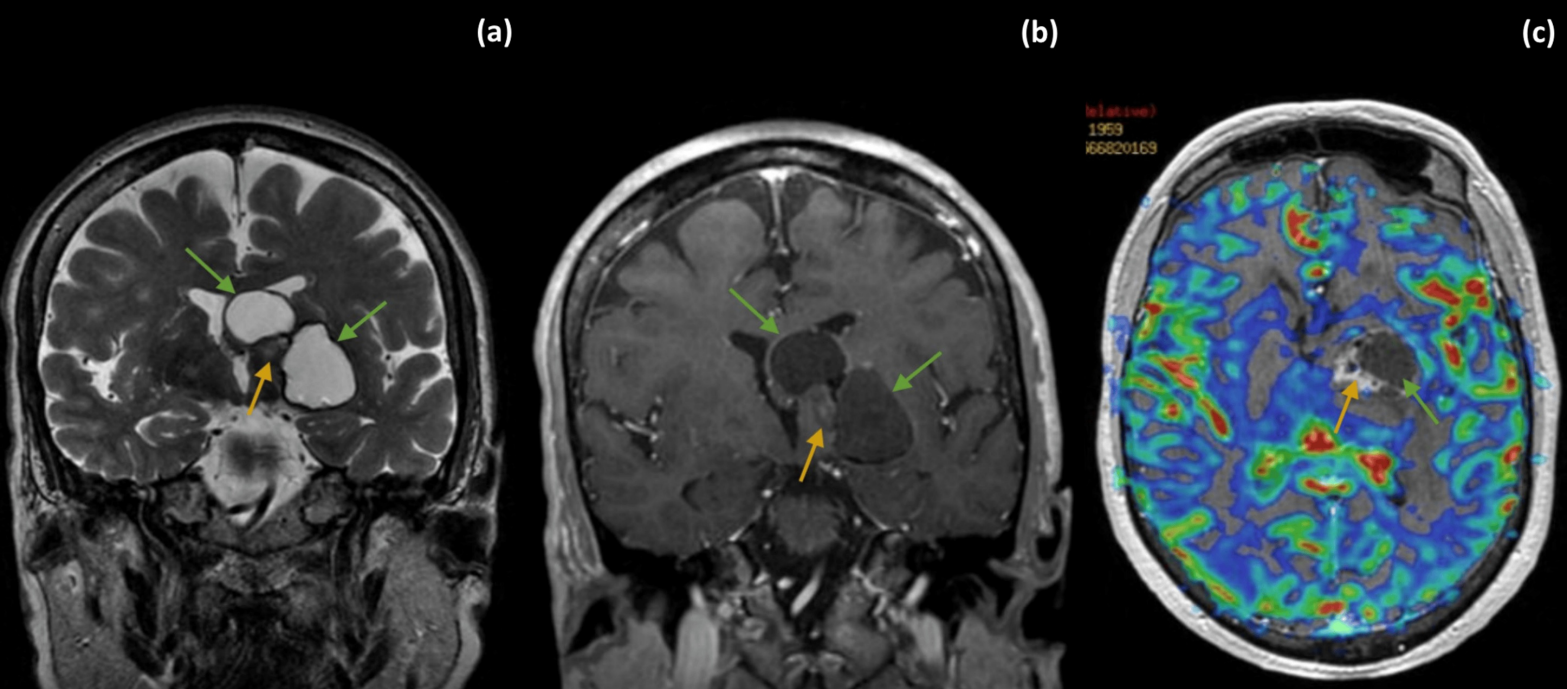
Diagnostic brain MRI. Brain MRI showed a left-side thalamic lesion with one solid and two large cystic components, one extending on the left lenticulocapsular region and the other encroaching to the body of the left lateral ventricle. The two cystic components (green arrows) were homogeneously hyperintense on T2-WI (a), with no contrast enhancement on post-contrast T1-WI (b). The solid component (yellow arrow) of the lesion showed mild heterogeneous enhancement on post-contrast T1-WI (b). On T2-WI (a), there is also visible discrete vasogenic edema in the parenchyma surrounding the lesion and right midline shift of the brain at the level of the septum pellucidum. There is no apparent increase in perfusion on the rCBV map (c). rCBV: relative cerebral blood volume
